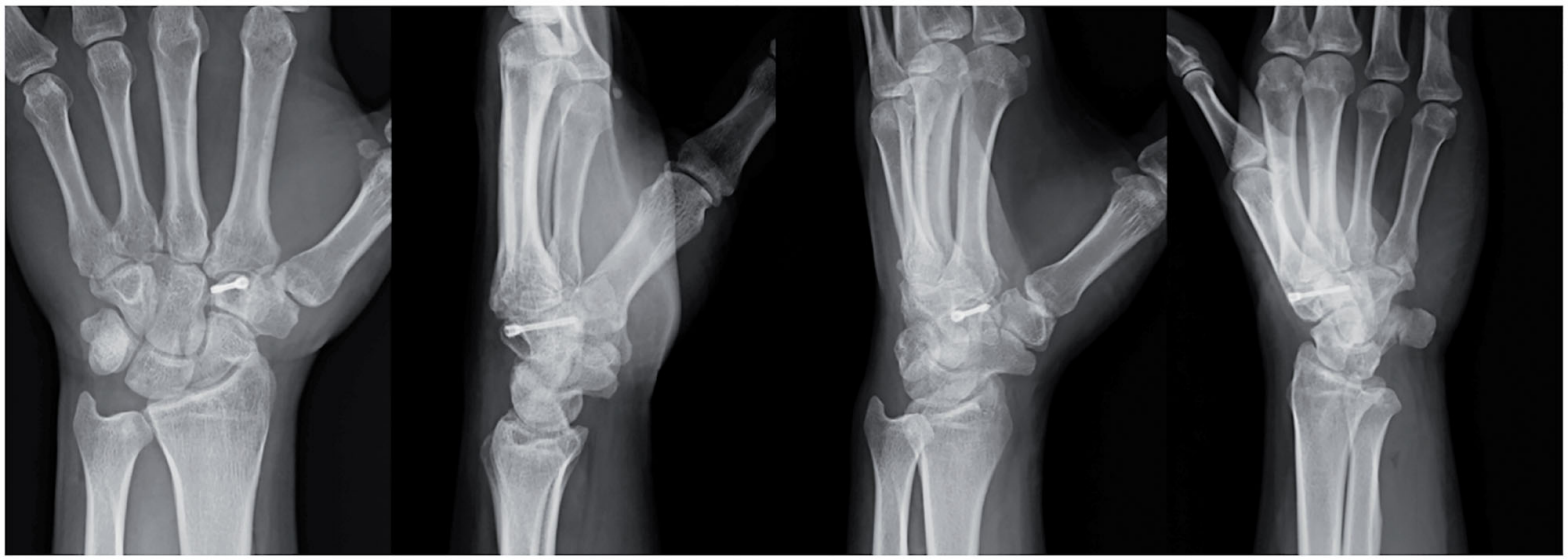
Postoperative X-ray images obtained at one year after surgical fixation of an isolated left trapezoid coronal shearing fracture in a 40-year-old male. Complete bone union and appropriate alignment of the proximal and distal carpal rows are visible. No arthritic changes of the second carpometacarpal joint or necrosis of the trapezoid can be seen.
